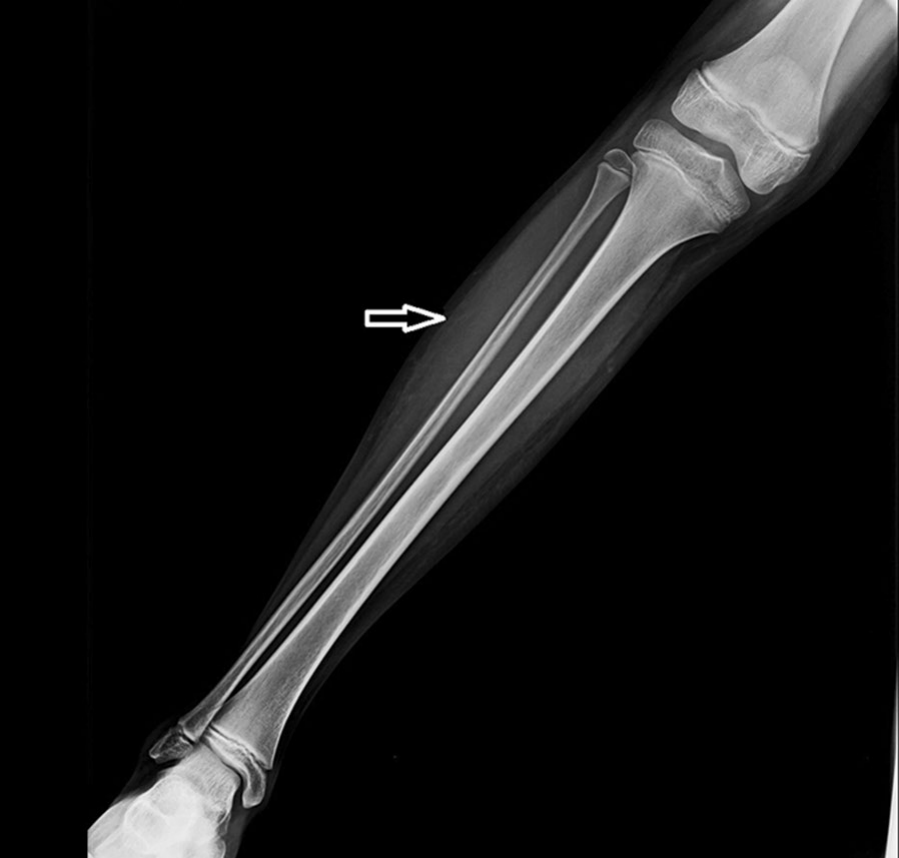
Plain radiograph anteroposterior projection demonstrating patchy irregular increased soft tissue densities in the lateral aspect of the middle third of the right leg as marked by the *arrow*
